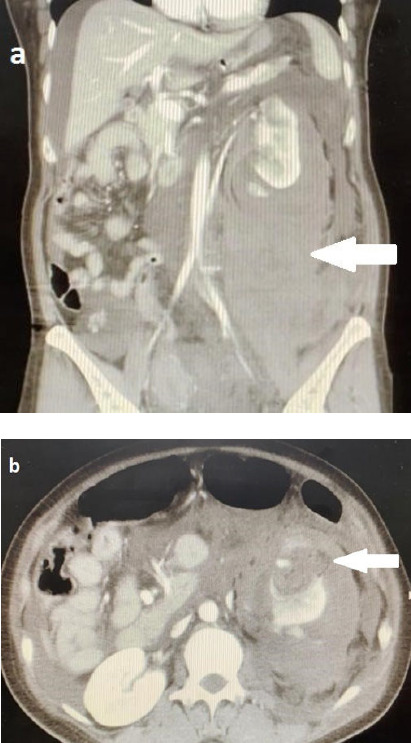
(a) Coronal CT image showing massive retroperitoneal haemorrhage mostly on the left side (arrow). (b) Axial CT image in the arterial phase showing a heterogeneous, mainly hypodense, mass at the lower pole of the left kidney suggestive of AML (arrow). AML, angiomyolipoma; CT, computed tomography
